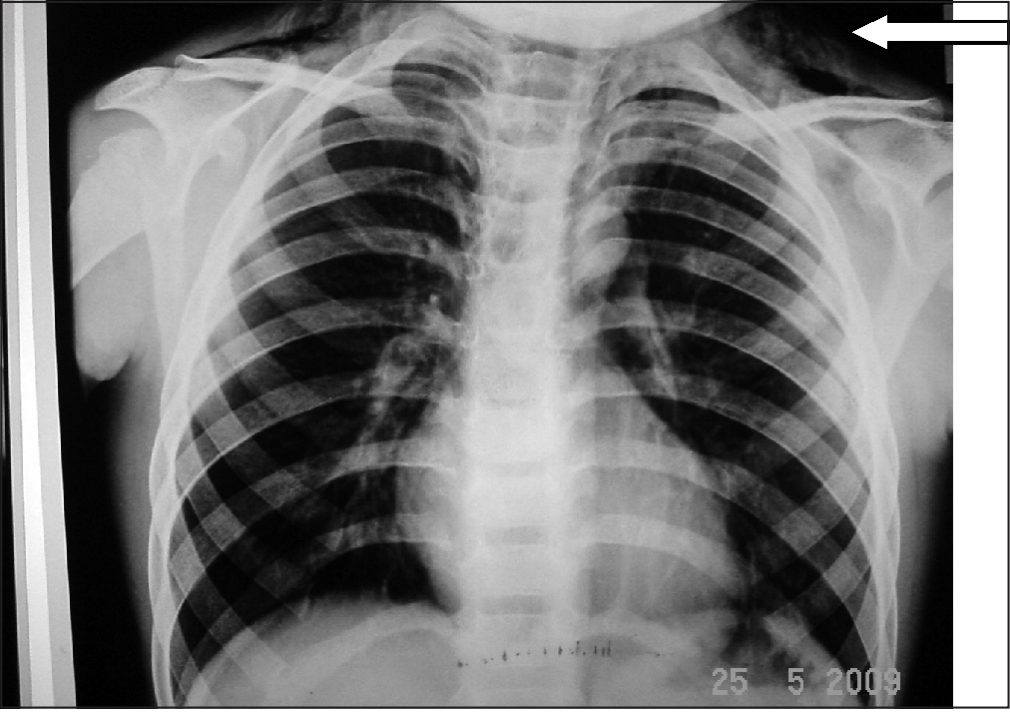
X-ray picture of the patient showing subcutaneous emphysema (marked by arrow) but no pneumothorax
